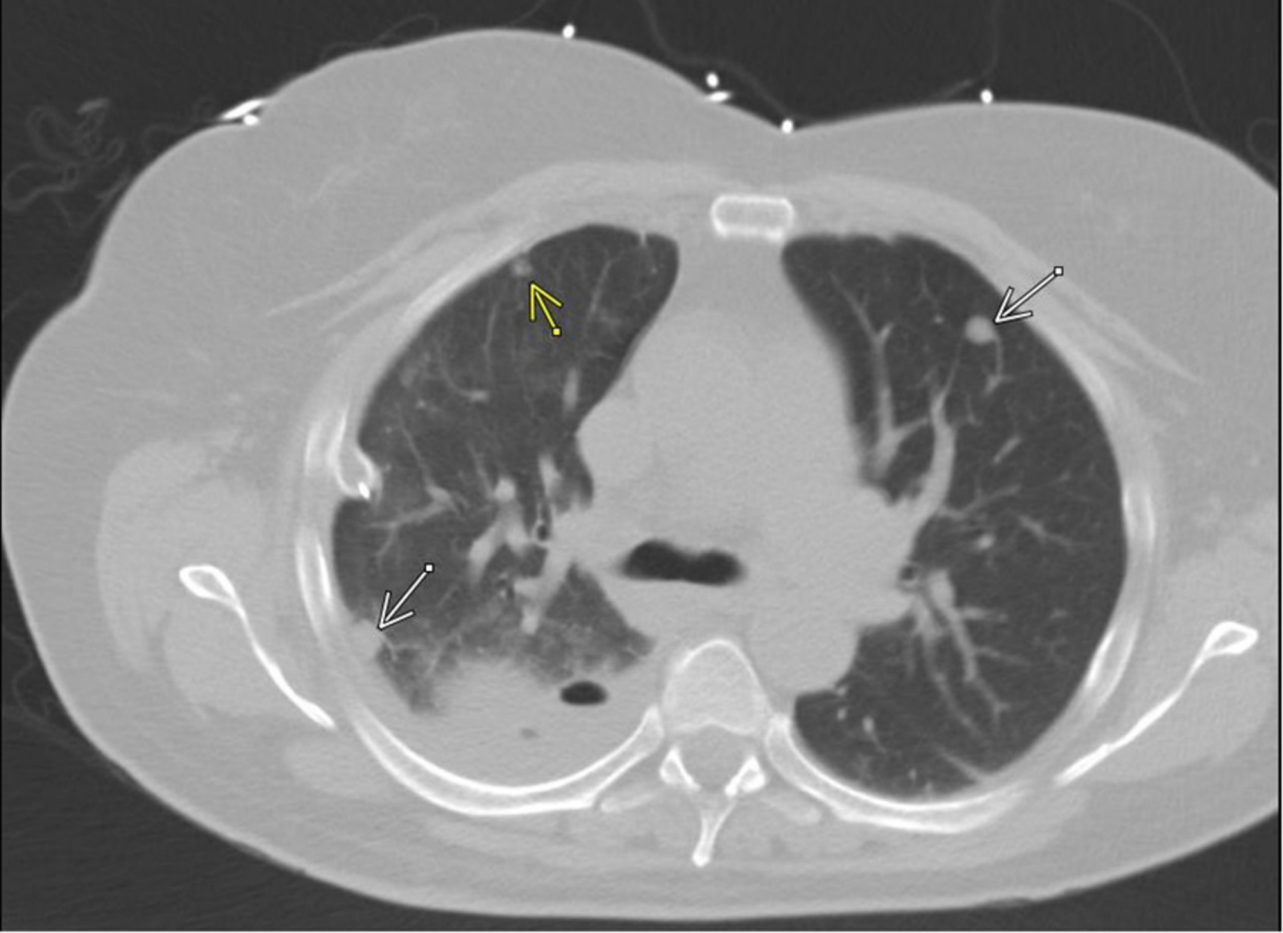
Chest CT scan 1/2015: Large right loculated pleural effusion, with multiple bilateral pulmonary nodules.
